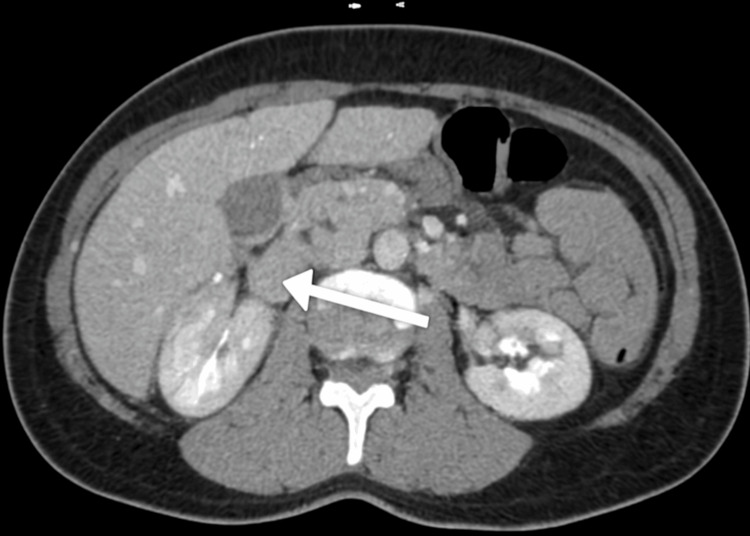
Axial CT scan of the abdomen revealing pancreatic edema An axial slice depicting the swollen pancreatic parenchyma (white arrow) with no signs of gallstones or biliary dilation.
